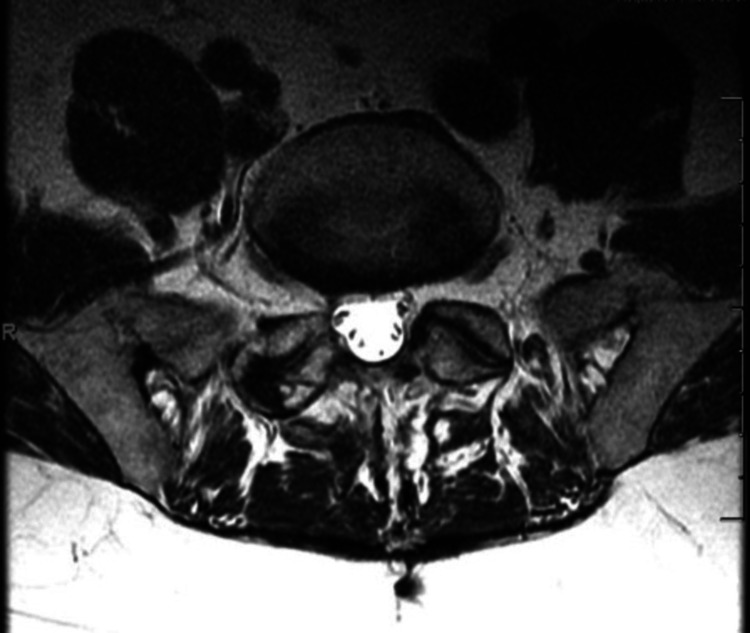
Axial view of the lumbar spine from a three-year post-operative MRI for Case 2.
